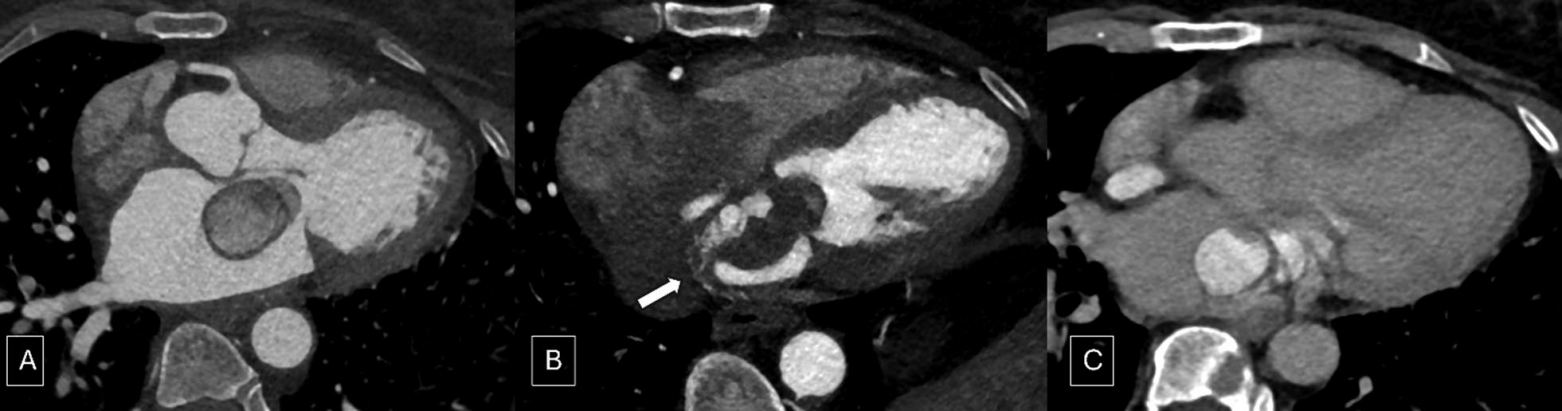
Cardiac CTA showing solid cystic enhancing mass lesion supplied from branches of left circumflex artery (A, B). On delayed images, progressive pooling of contrast was seen within cystic spaces
